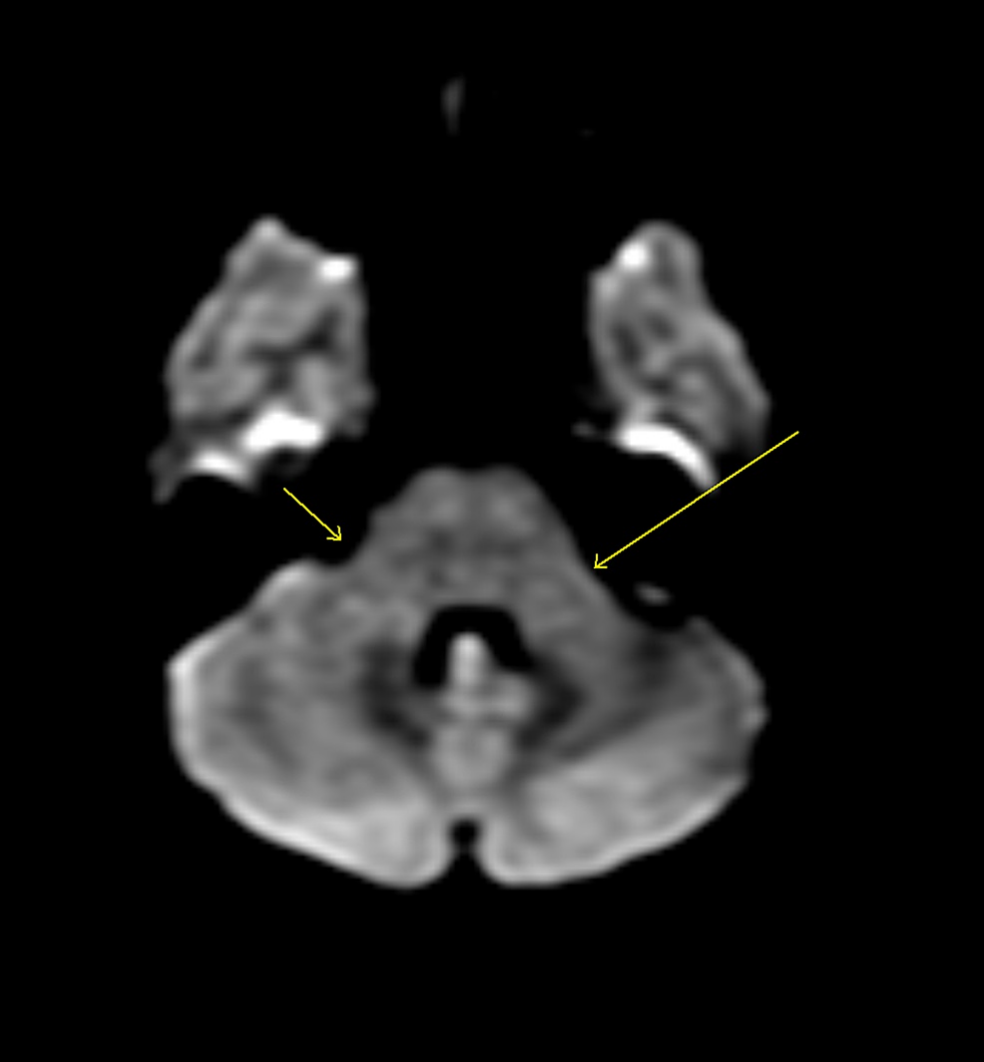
Diffusion-weighted MRI, post-contrast phase. The arrows show marked improvement in the brachium pontis lesion.
